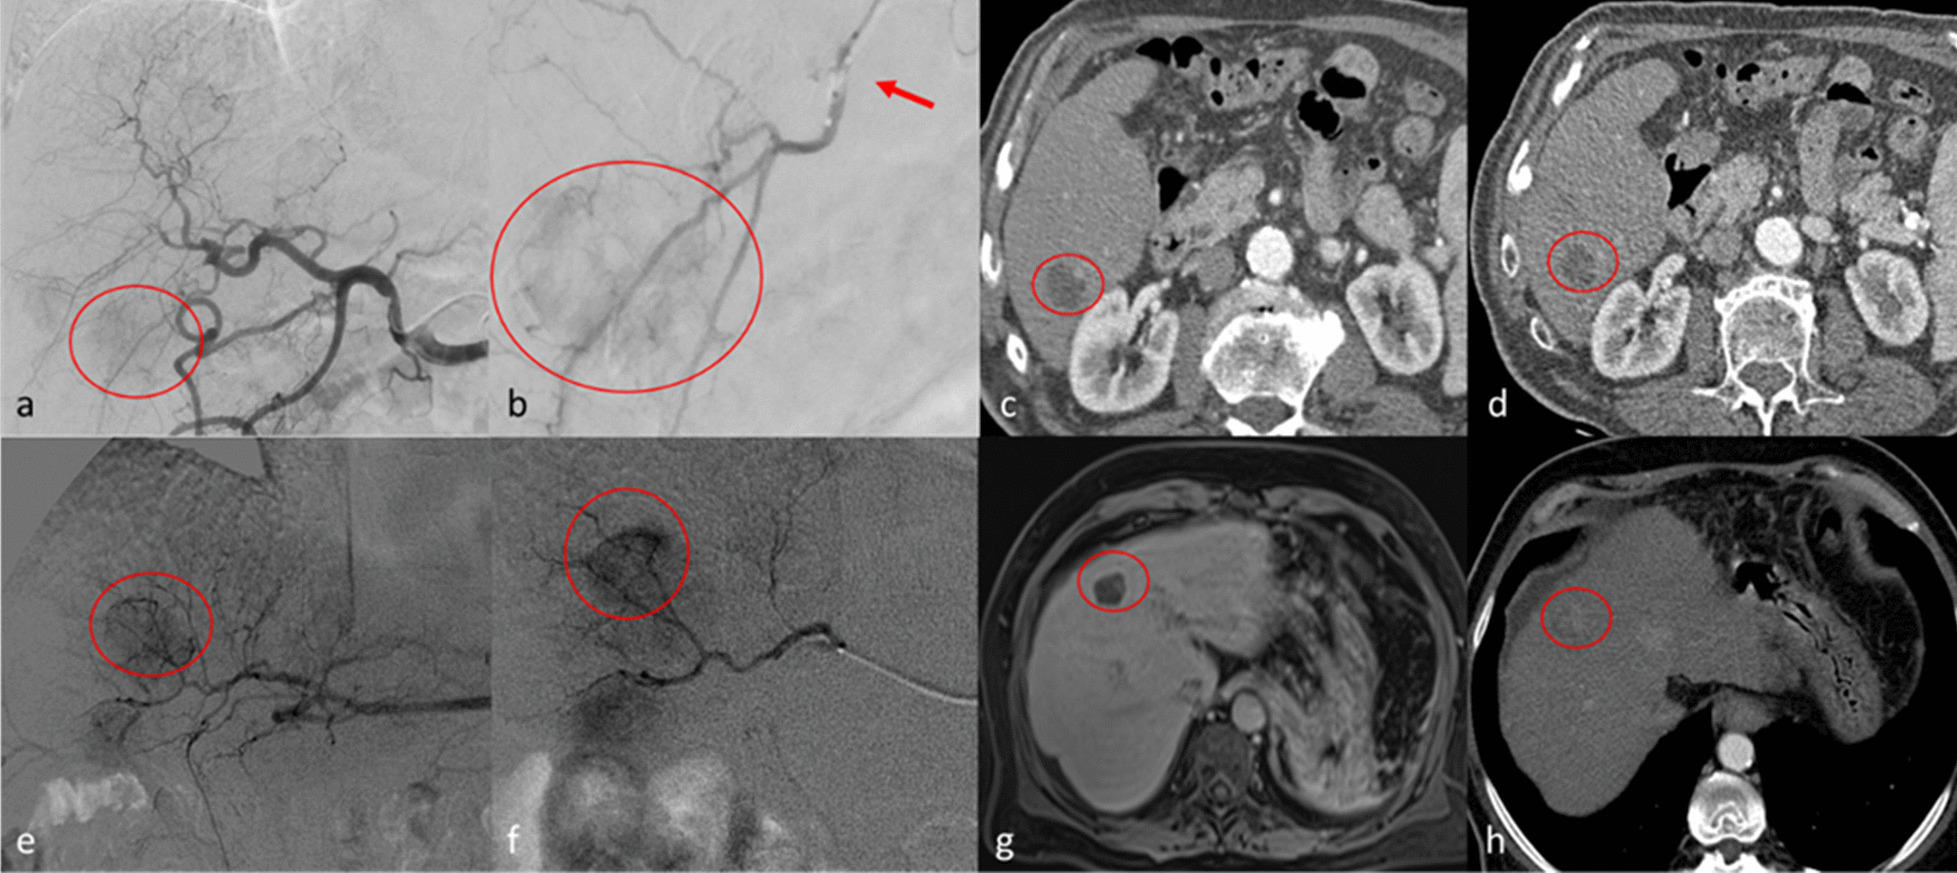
Top row. Clinical case of a 54 years old male with hepatocellular carcinoma [HCC] (diameters: 25 × 23 mm) in segment 6. a digital subtraction angiography (DSA) obtained with injection from common hepatic artery demonstrates a hyper vascular tumor (circle); b selective DSA with inflated balloon micro-catheter (arrow) confirms the HCC (circle). c, d show the arterial phase of contrast enhanced computed tomography which demonstrate complete response at 1 month (c) and persisting complete response at 6 months (d). Bottom row. Clinical case of a 61 years old female with HCC (diameters: 22 × 21 mm) in the segment 4. e, f DSA from common hepatic artery and super-selective DSA with micro-catheter, respectively, demonstrate the HCC (circle). g MR imaging follow-up in hepatobiliary phase shows complete response at 1 month (circle); h at 6 months follow-up, computed tomography in arterial phase shows only a partial response
